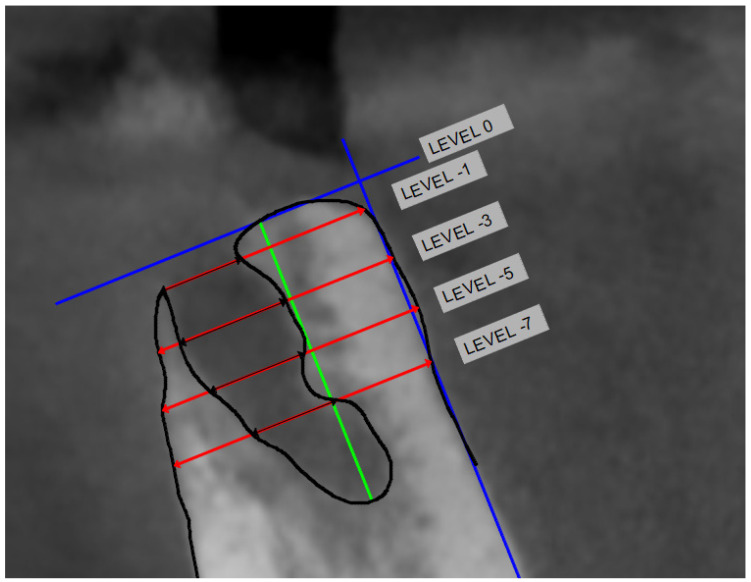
The cross-sectional CBCT slide pertaining to the center of the extracted root(s) were selected for measurement. First, most coronal bone was identified with a line perpendicular to the long axis of the lingual bone (blue line). Then, 4 lines were constructed perpendicular to this axis at the following levels: −1 mm apical to the most coronal bone line (L1), −3 mm apical to the most coronal bone line (L3), −5 mm apical to the most coronal bone line (L5), and −7 mm apical to the most coronal bone line (L7). Vmax is the distance from L0 to the most apical part of the defect (green line).
